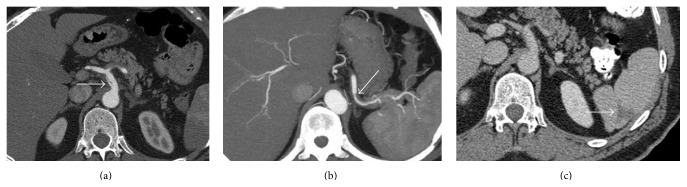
Axial enhanced CT scan shows (a) an intimal dissection of the celiac artery with opacification of true and false lumen, (b) a dissection membrane in the splenic artery causing poor contrast flow and thrombosis of the false lumen, and (c) a posterolateral splenic infarction.
